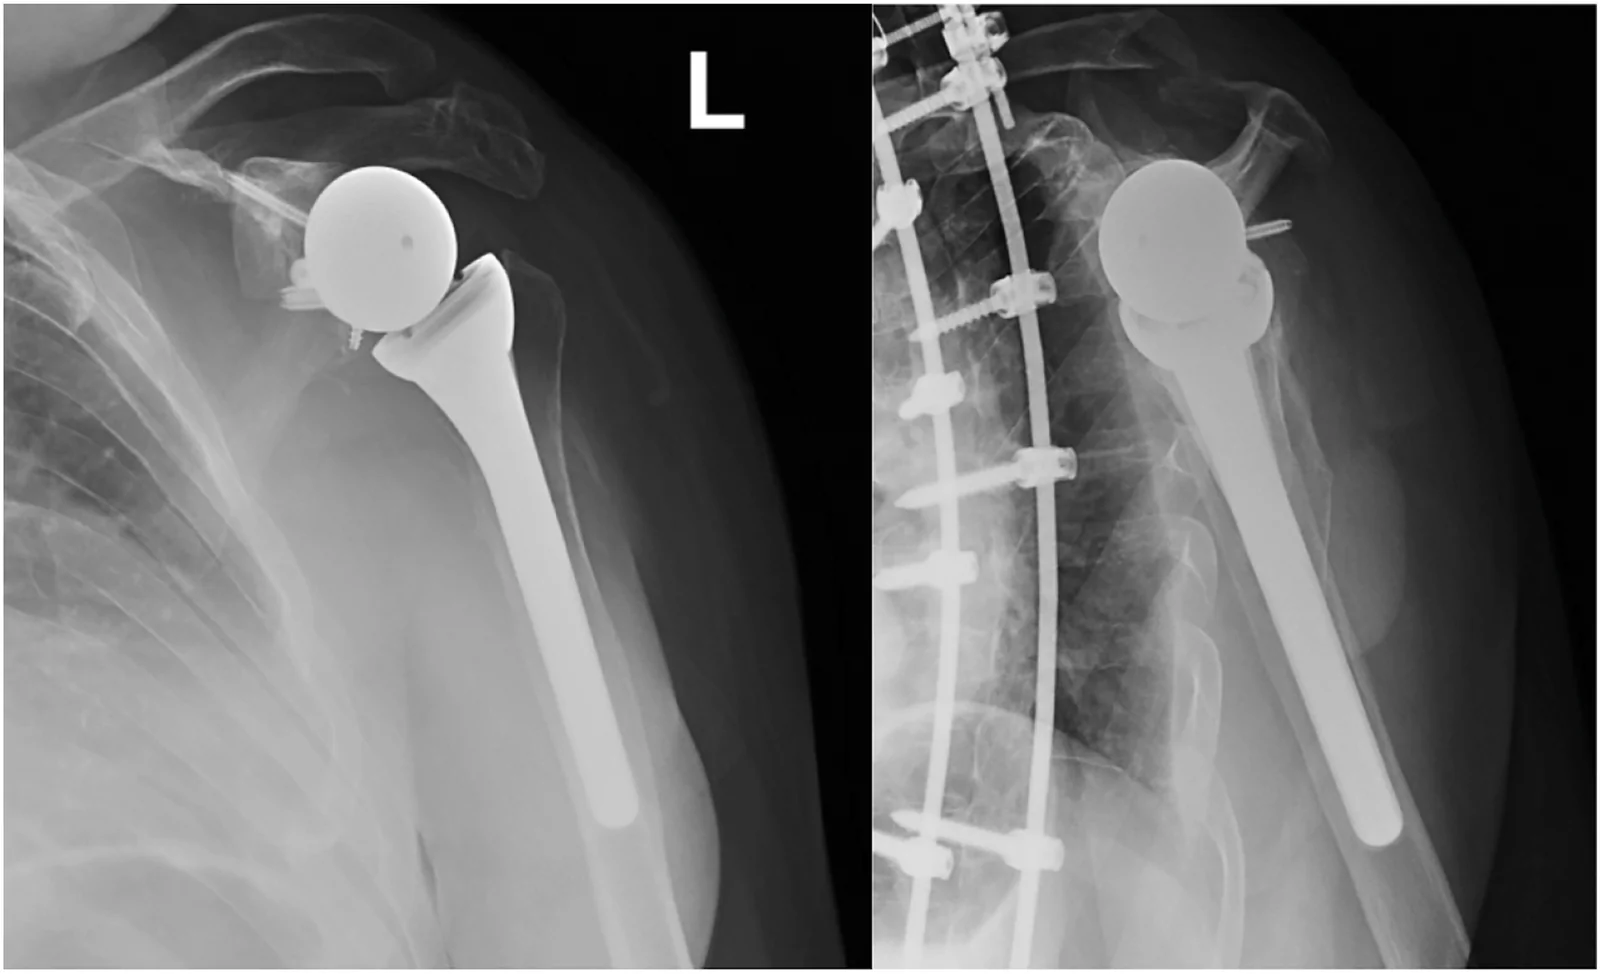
Case 2 x-rays at 3-year follow-up.
